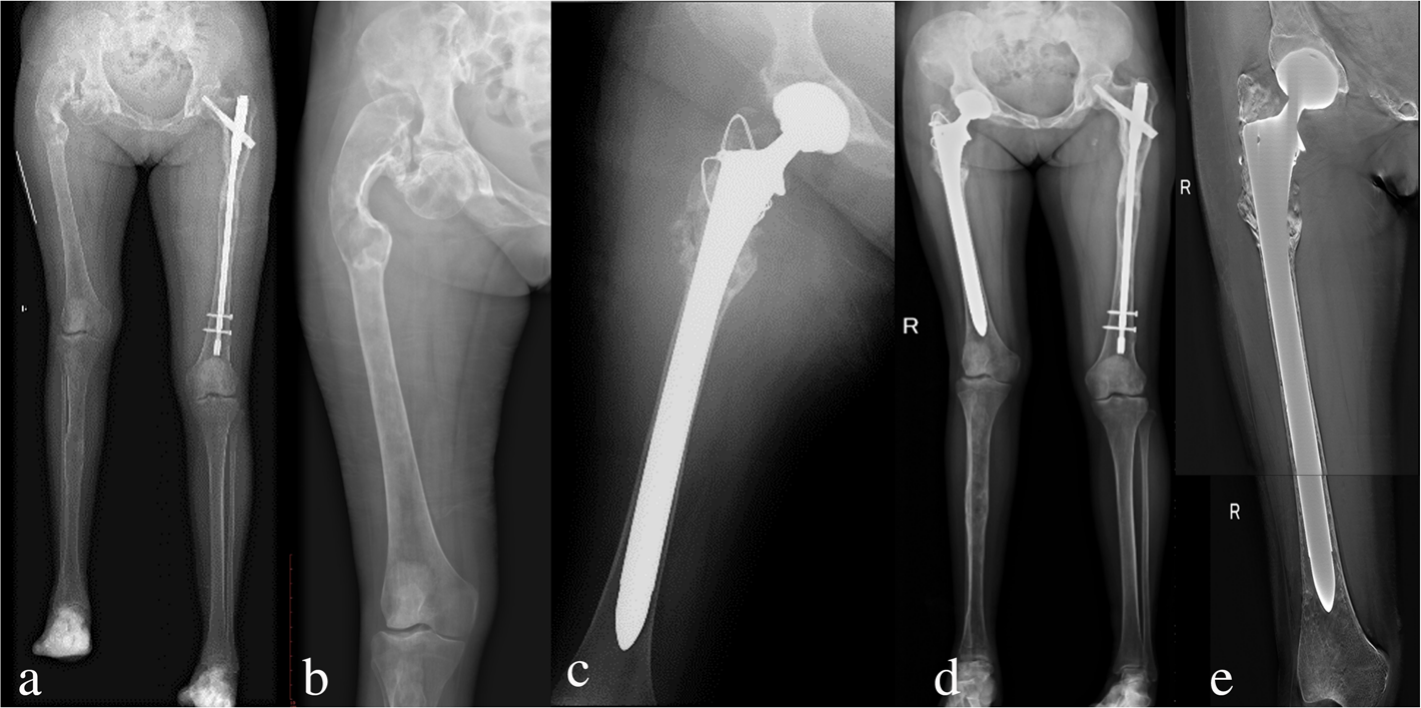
Preoperative radiography (a, b), postoperative radiography (c), and at the 36 months follow-up of radiography (d) and T-SMART image (e) in patient 9
